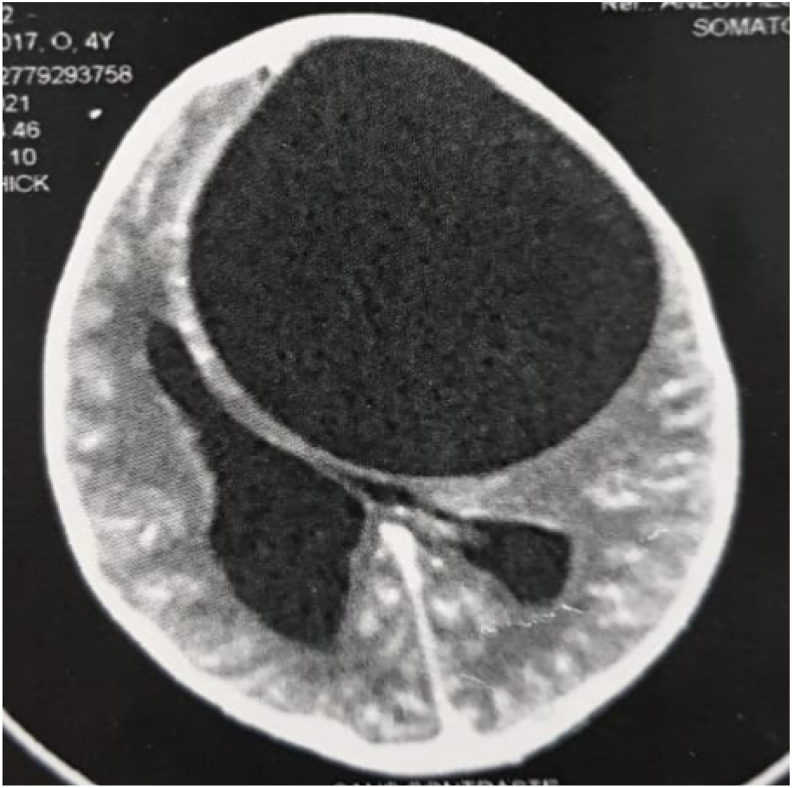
A brain CT scan was performed, showing a round hypodense intra-parenchymal lesion at the left fronto-parietal level with an important mass effect on the midline.
